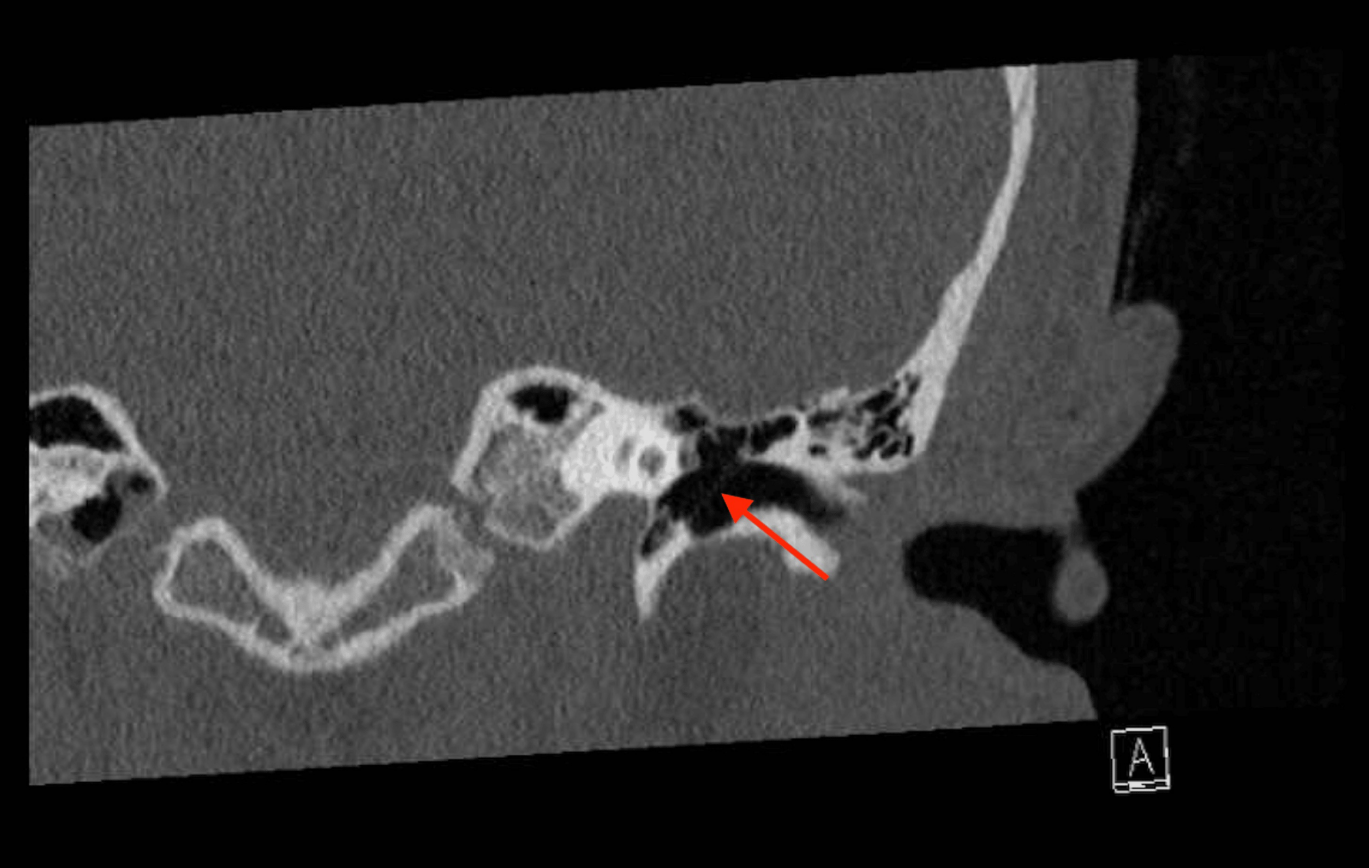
Coronal view of a temporal CT scan without contrast, displaying the left internal acoustic canal with a thickened left tympanic membrane (indicated by a red arrow)
